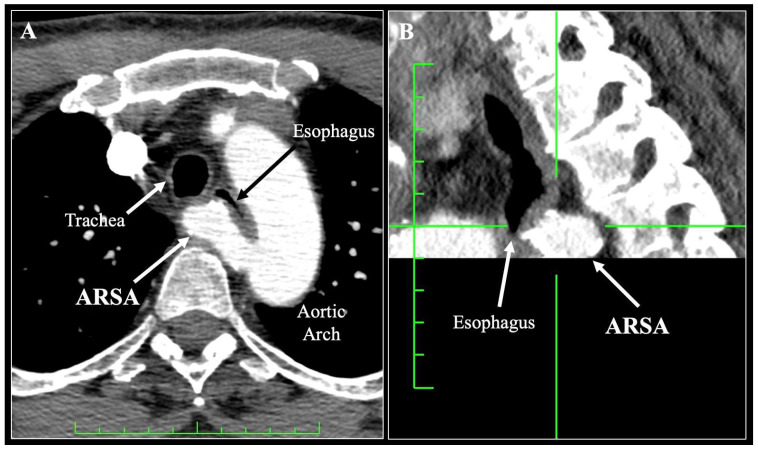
Computed tomography angiography of an aberrant right subclavian artery (ARSA) coursing posterior to the esophagus demonstrated in axial (A) and sagittal (B) reconstructions.
